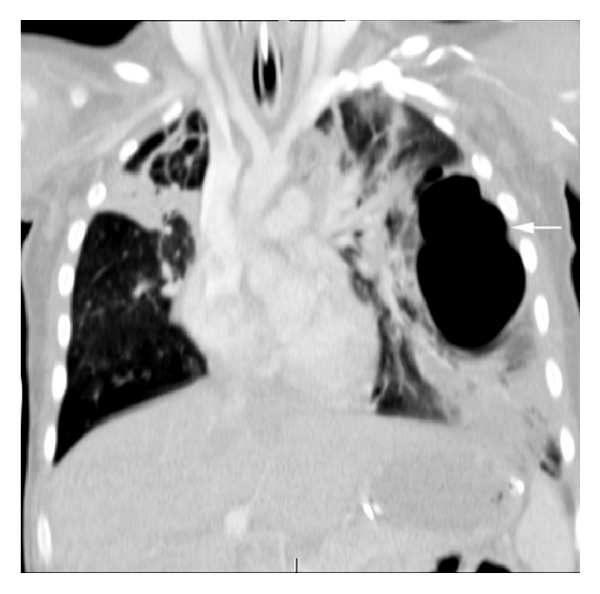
Coronal view on CT chest: a large and irregular pneumatocele extending throughout the left lung with extensive pulmonary contusions.
